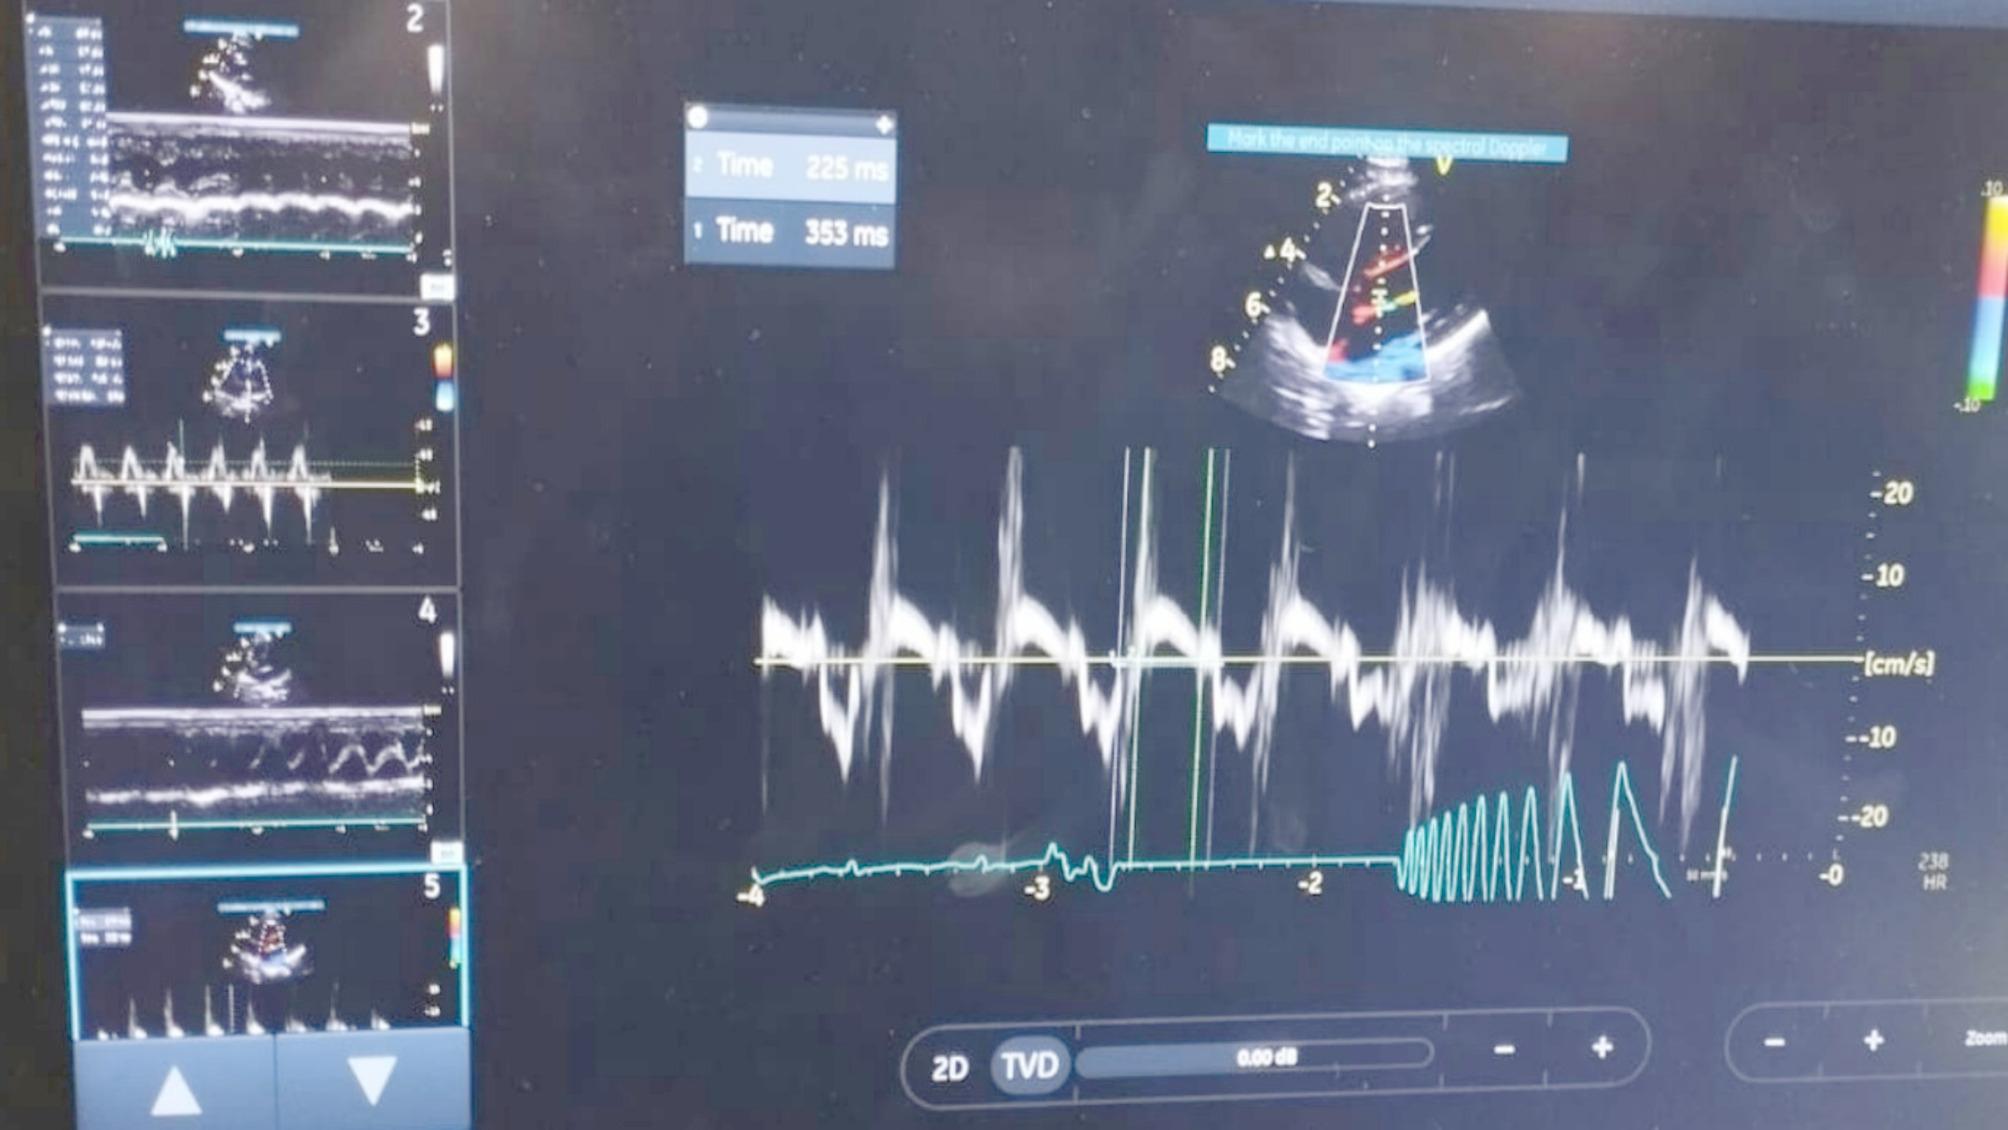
Pulsed Wave Tissue Doppler Imaging Before Treatment. Pulsed wave tissue Doppler imaging from the apical 4-chamber view, sampling from the septal mitral annulus before treatment. The E’/A’ ratio is 0.8, and the left ventricular myocardial performance index (LV MPI) is 0.56 (353 − 225/225). This indicates impaired myocardial relaxation and systolic dysfunction, characteristic of patients with Sanfillipo MPS 3
